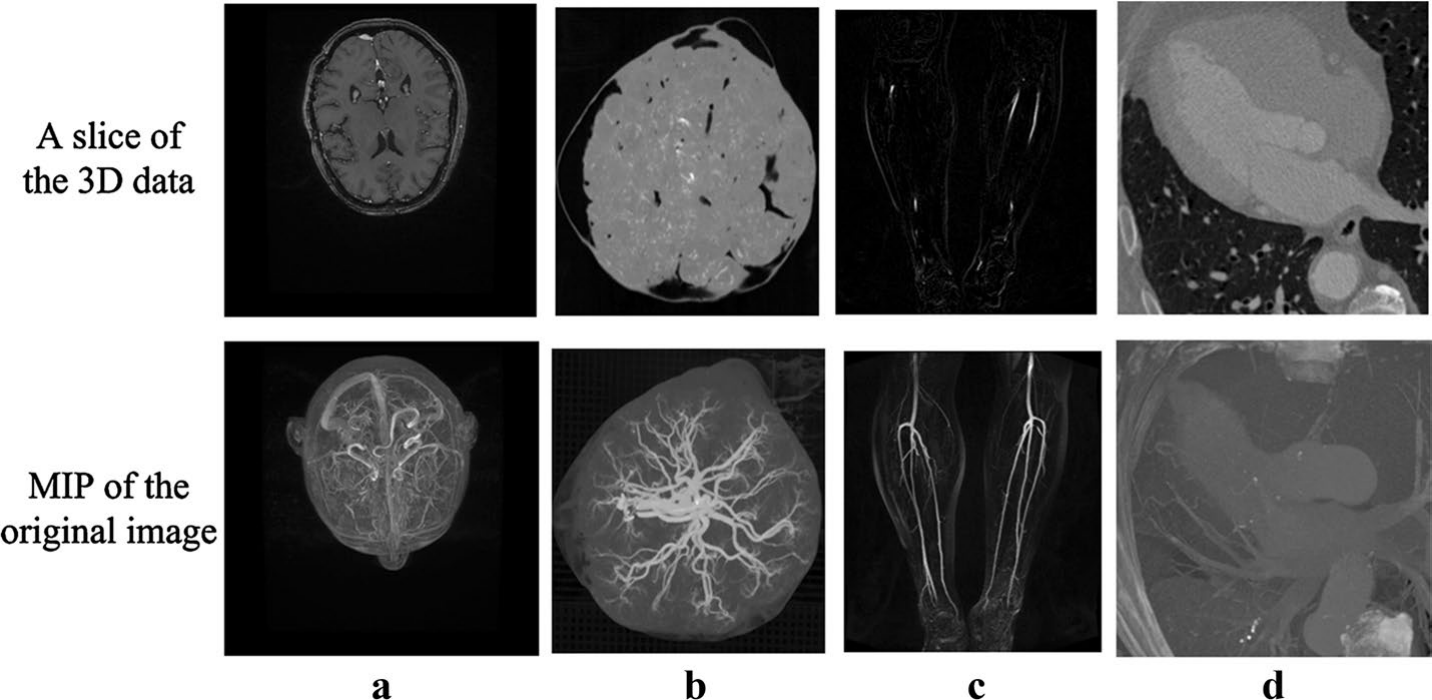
The clinical data sets. a Brain MRA data. b Placenta MRA data. c Legs MRA data. d Thorax CTA data
